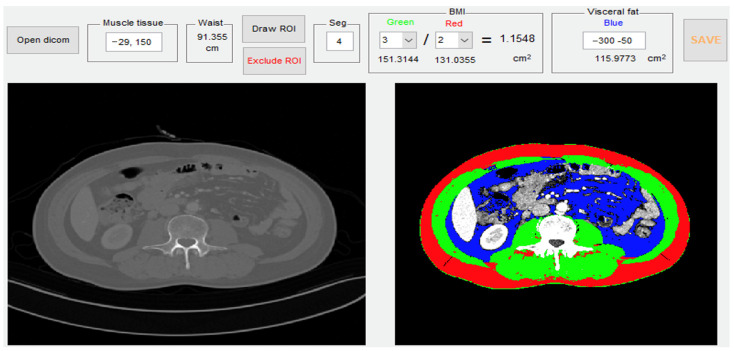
The measurements of body composition using abdominal CT scan image. SMA (green, cm^2^), SFA (red, cm^2^), and VFA (blue, cm^2^). MATLAB version R2014a (Mathworks Inc., Natick, MA, USA) (https://sourceforge.net/projects/muscle-fat-area-measurement (accessed on 1 September 2023)).
